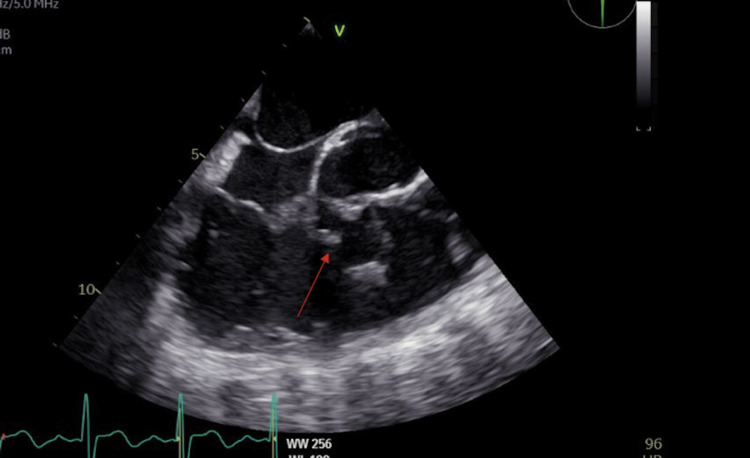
TEE four-chamber view of the VSD patch. TEE, transesophageal echocardiogram; VSD, ventricular septal defect
